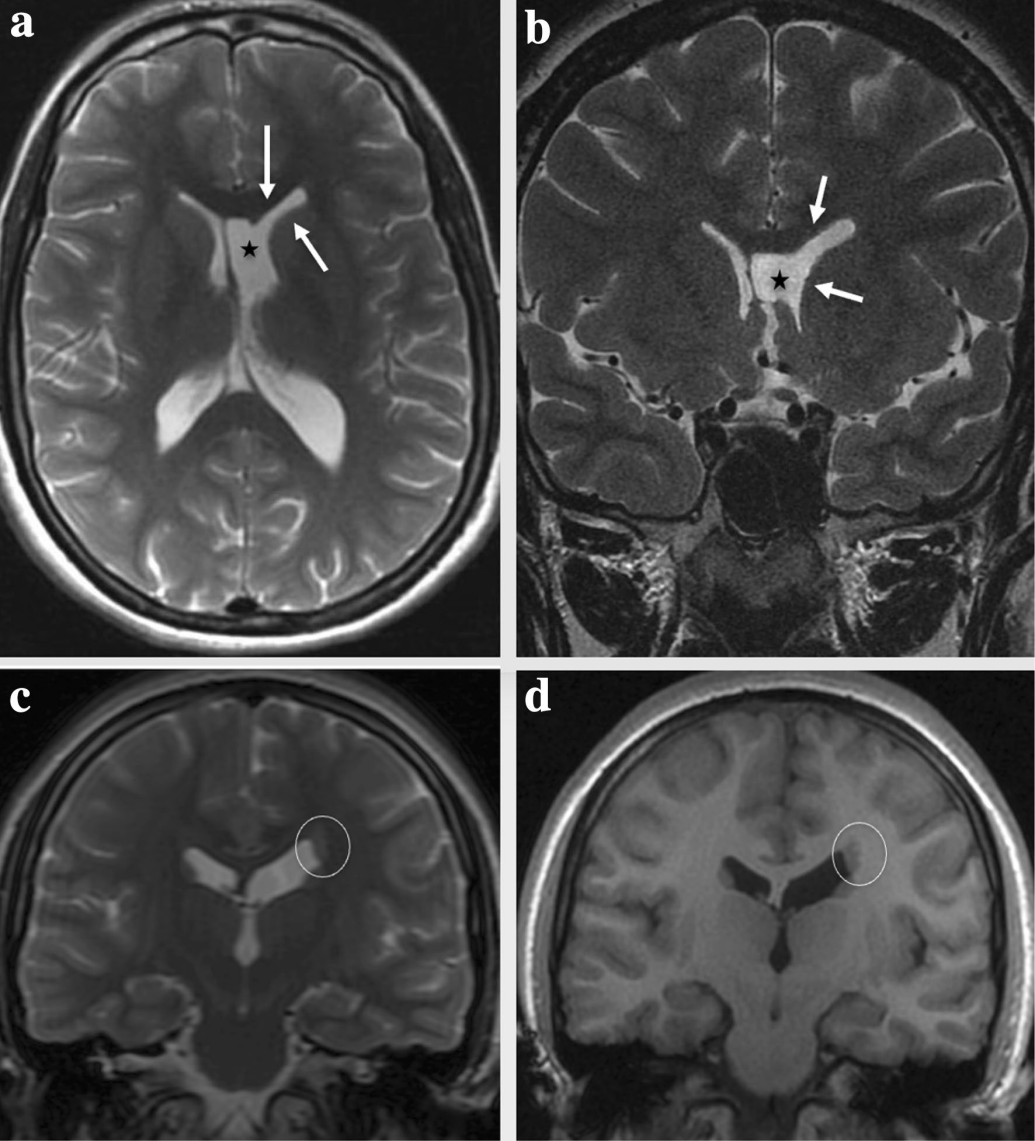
(ID 109): Dysmorphic left lateral ventricles and periventricular nodular heterotopia (PVNH)*. a, b Unenhanced axial T2-weighted (T2w) magnetic resonance image a and unenhanced coronal T2-weighted (T2w) magnetic resonance image b shows dysmorphic left lateral ventricles (white arrows) that appear fused with cyst of the cavum septum pellucidum (black star). c, d Coronal T2-weighted (T2w) magnetic resonance image c and T1 3D FSPGR (fast spoiled gradient echo) coronal magnetic resonance image d shows periventricular nodular heterotopia (white circle)
